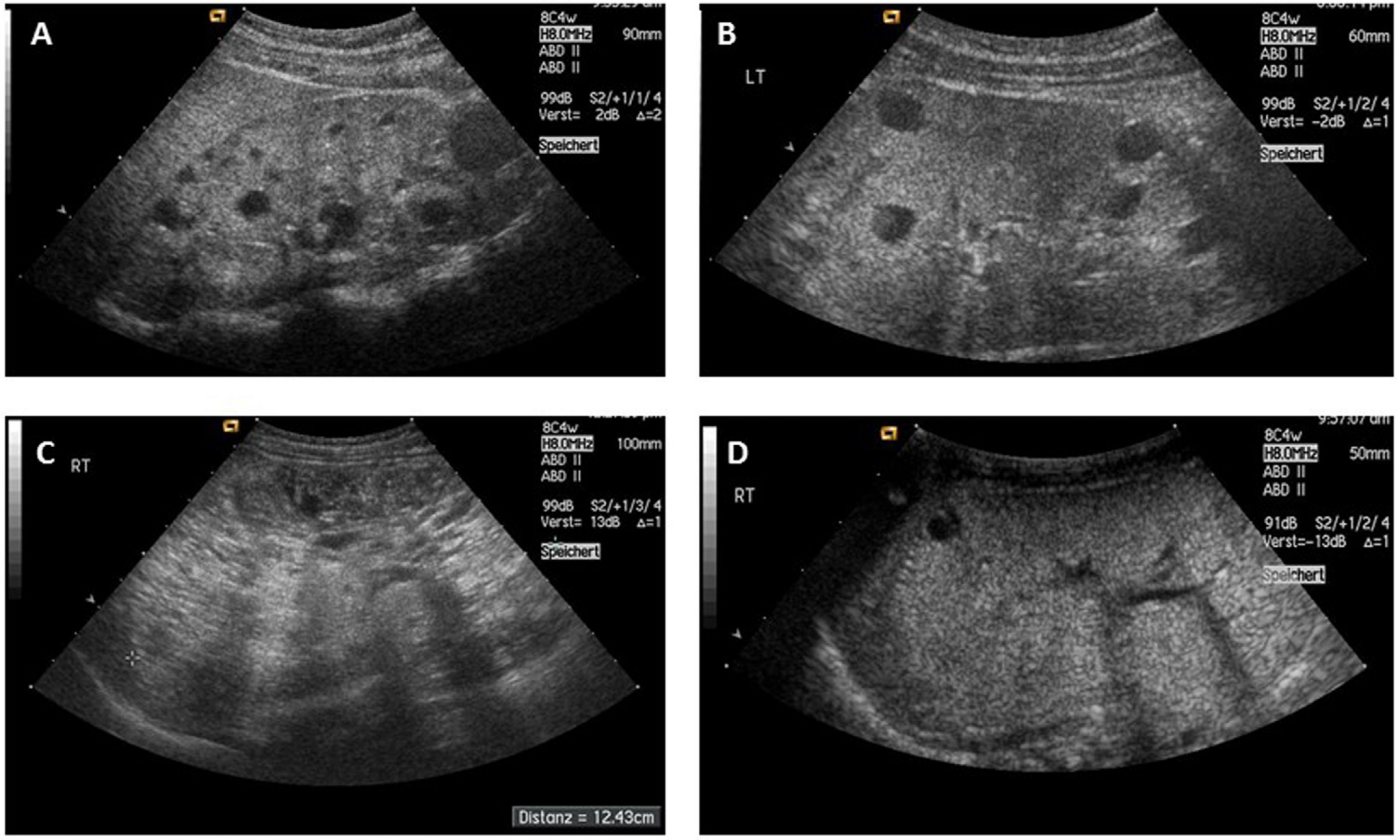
(A,B) Typical sonographic picture of ADPKD in a 10-month-old girl (A) and an 11-year-old boy (B). (C) ADPKD resembling ARPKD in a 3-year-old boy with enlarged echogenic kidneys and small sized cysts. (D) Three-week-old girl with previous oligohydramnion, arterial hypertension, and small cysts in massively enlarged kidneys with a calculated total kidney volume of about 100 ml (normal age-related value <40 ml). Her mother’s phenotype resembled ADPKD, but the family was shown to carry an *HNF1β* germline mutation [from Bergmann (5)].
